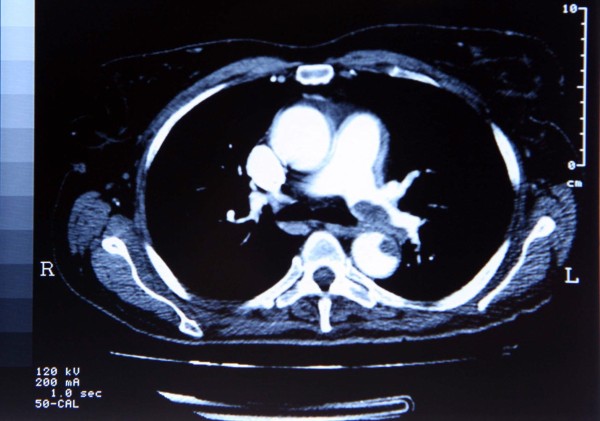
A computed tomography slice of the thorax showing a mural aortic thrombus in the anterior wall of the descending aorta and a mural thrombus in the left pulmonary artery localized in close proximity to the aortic thrombus.
